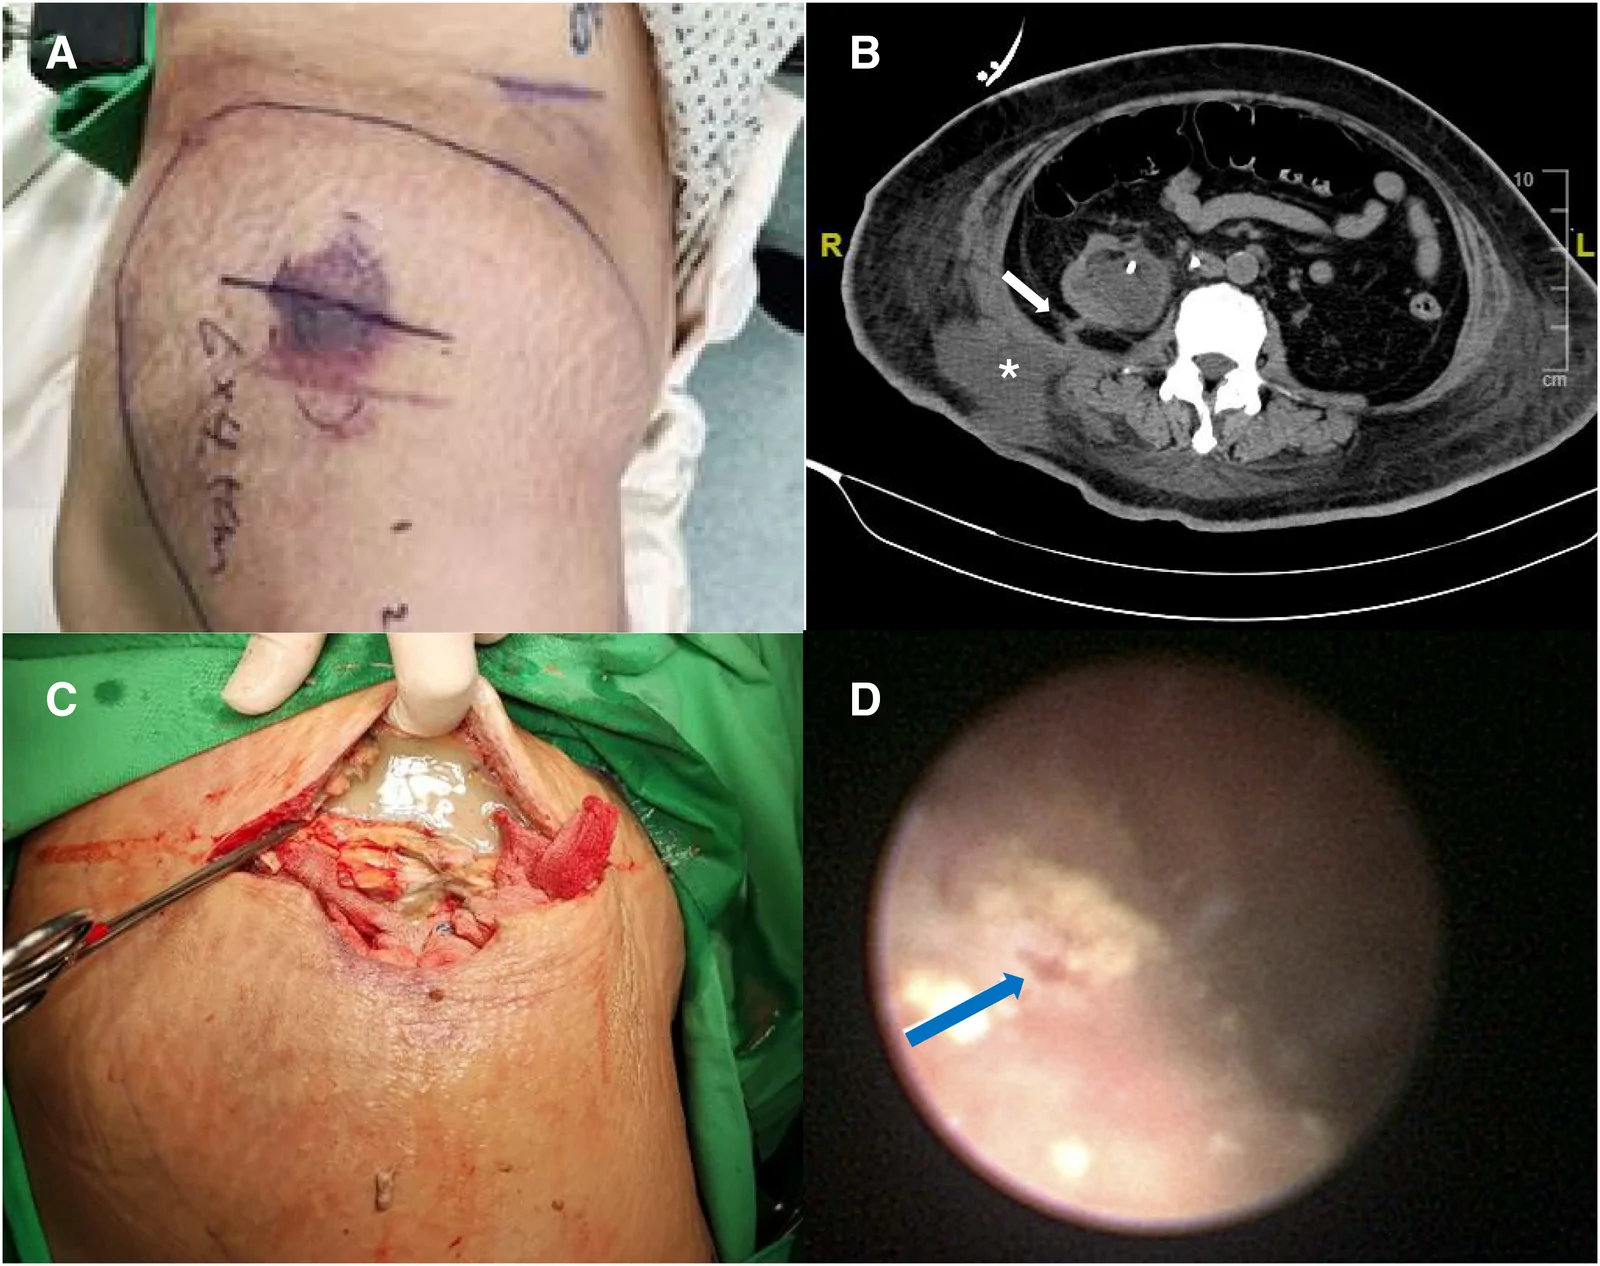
Findings 2 years after the prior emphysematous pyelonephritis episode. (A) Clinical photograph showing right hip induration, skin discoloration, tenderness, and crepitus. (B) Non-contrast CT scan demonstrating a posterolateral abdominal wall abscess (asterisk) with a suspected tract connecting to the right kidney (arrow), consistent with a delayed infectious extension. (C) Intraoperative findings revealing extensive pus accumulation and free air within the muscular fascia during surgical exploration. (D) Cystoureteroscopy revealing yellowish-white encrustation with stone formation at the right side ureter and a mucosal defect (arrow) which might be a fistula. CT = computed tomography.
